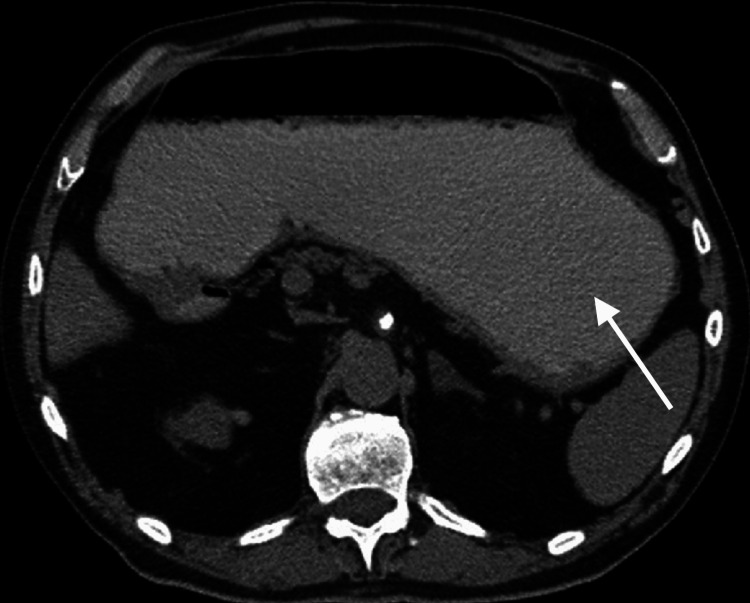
CT scan of abdomen indicating delayed gastric emptying.
